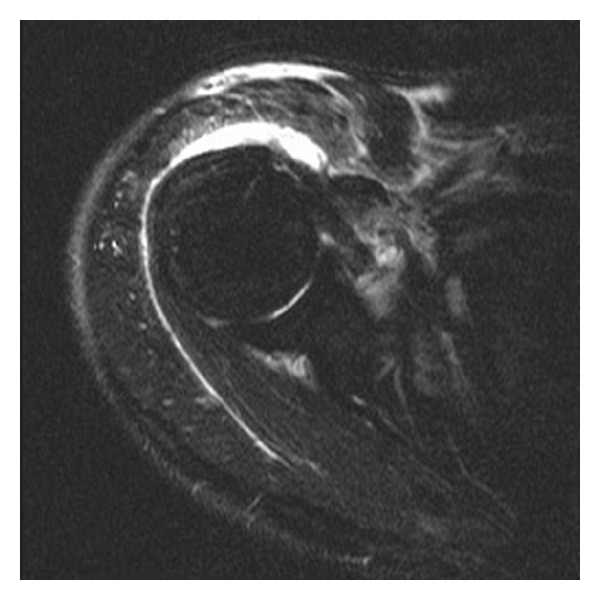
Axial T2-weighted MRI scan showing collection in anterior aspect of subacromial bursa.
